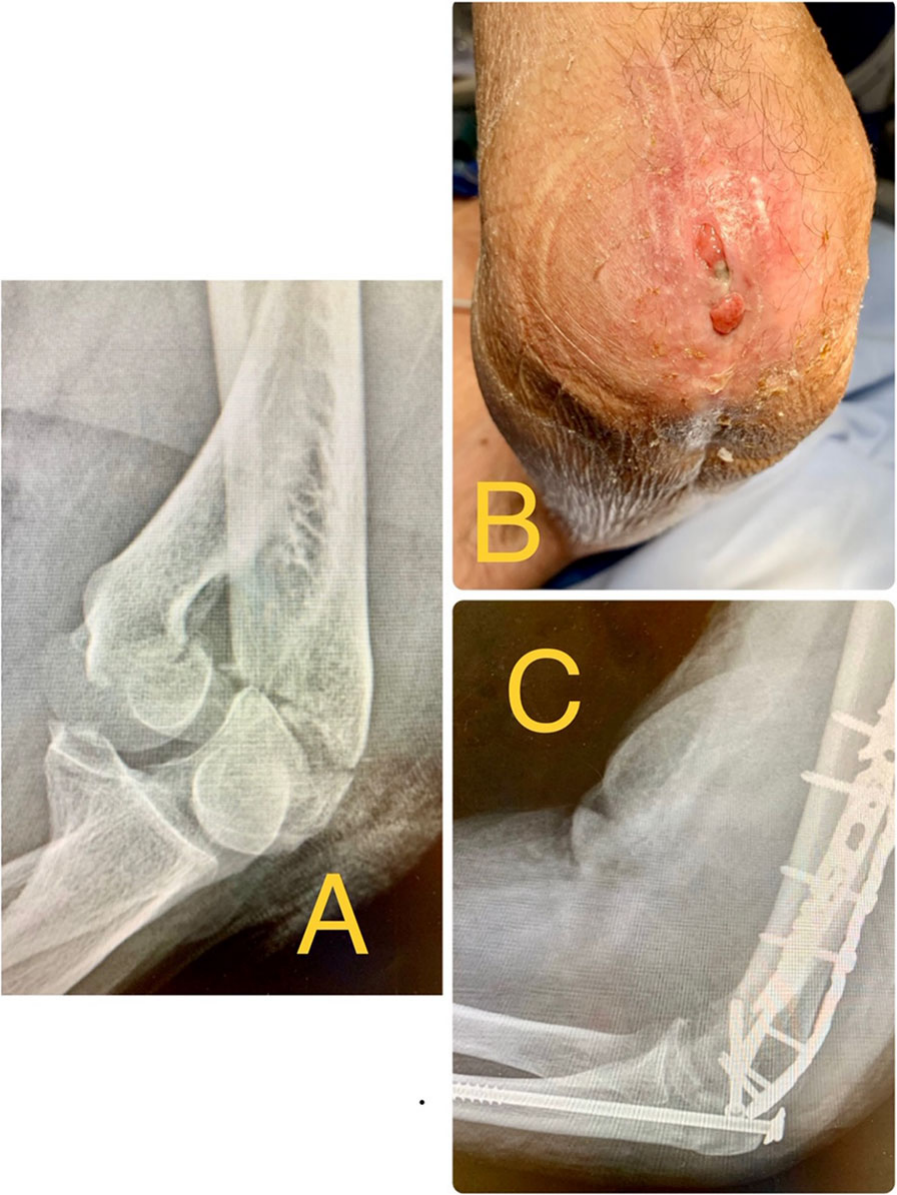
a 83 year old man who presented a conminuted supraintercondylear fracture of the humerus. b Wound dehiscence that required outpatient nursing cures every 48 h until enough bone callus was observed in most part of the ulna after 10 weeks; the wound healed well after the screw removal. c X-ray at 10 weeks after the first surgery. Osteosynthesis of the fracture with two plates and a 6.5 mm thick and 115 mm long screw with washer for the olecranon osteotomy. Enough bone callus was observed in most part of the ulna, considering the bone union stable to remove the screw
